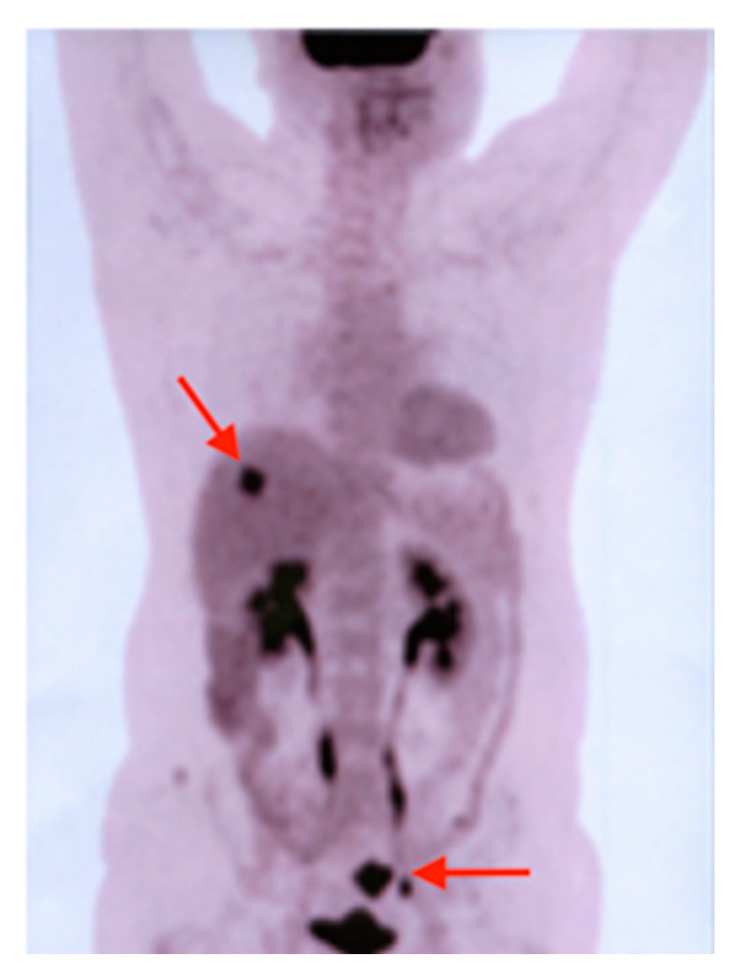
MIP image of F18-FDG PET-CT revealing a lesion in the sigmoid colon (lower-right red arrow) and a lesion of 21 mm in the VIIth liver segment (upper-left red arrow), consisting of a colon malignant tumor with liver metastases.
